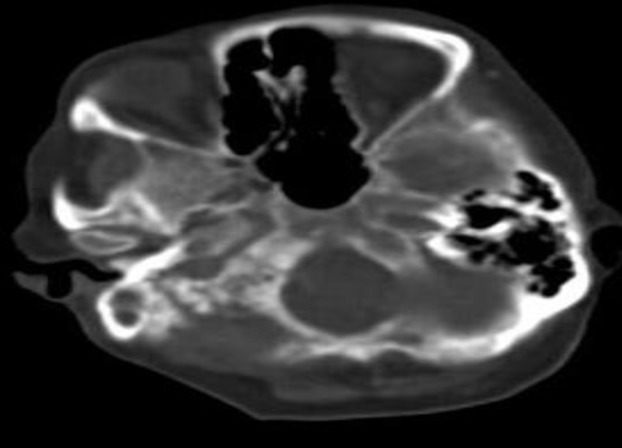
CT temporal bone showing a soft tissue density lesion in the right mastoid air cells with sclerosis. The sclerosis extends into the basiocciput and lateral part of the clivus
